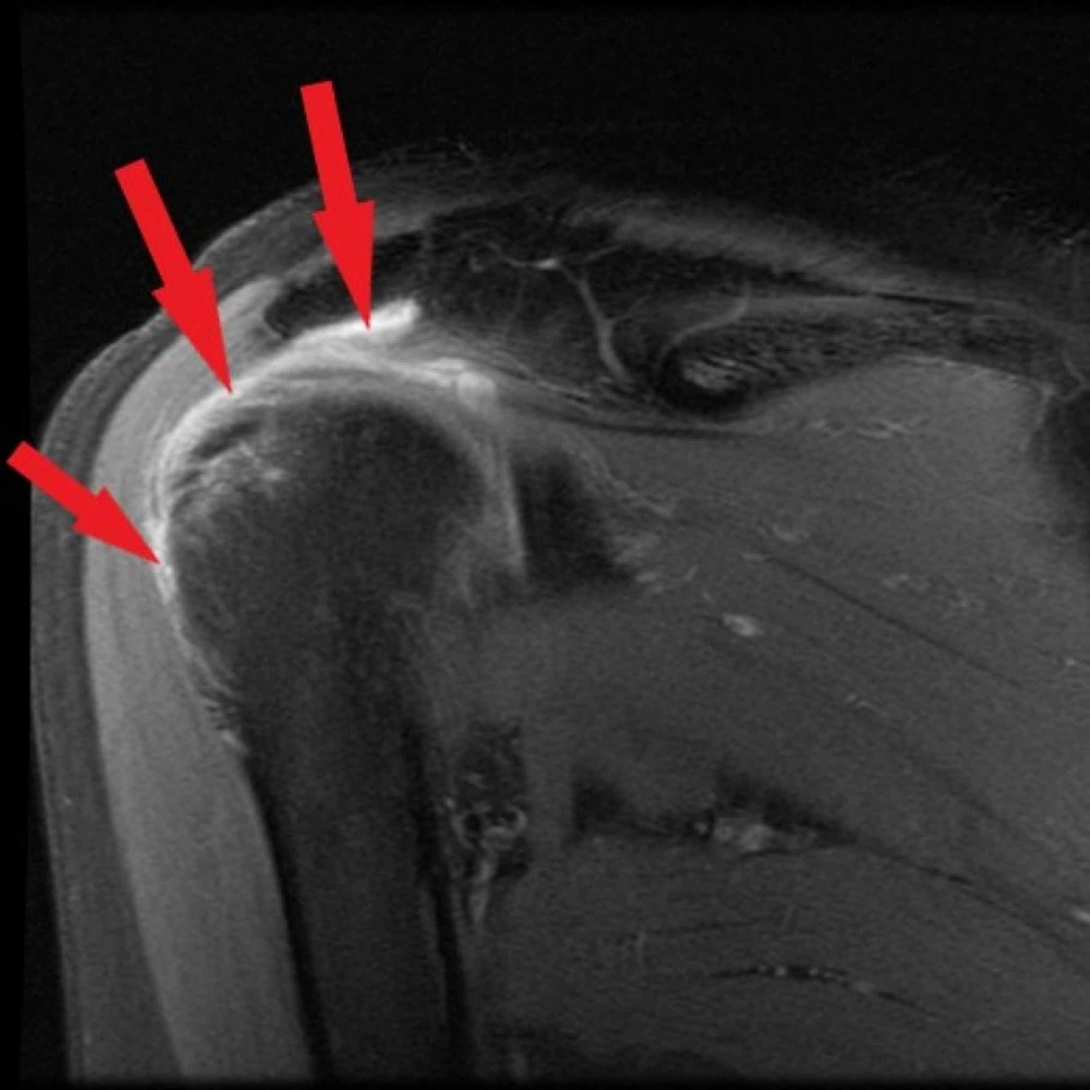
MRI left shoulder MRI left shoulder with arrows showing excess fluid in the subdeltoid bursae indicating bursitis
